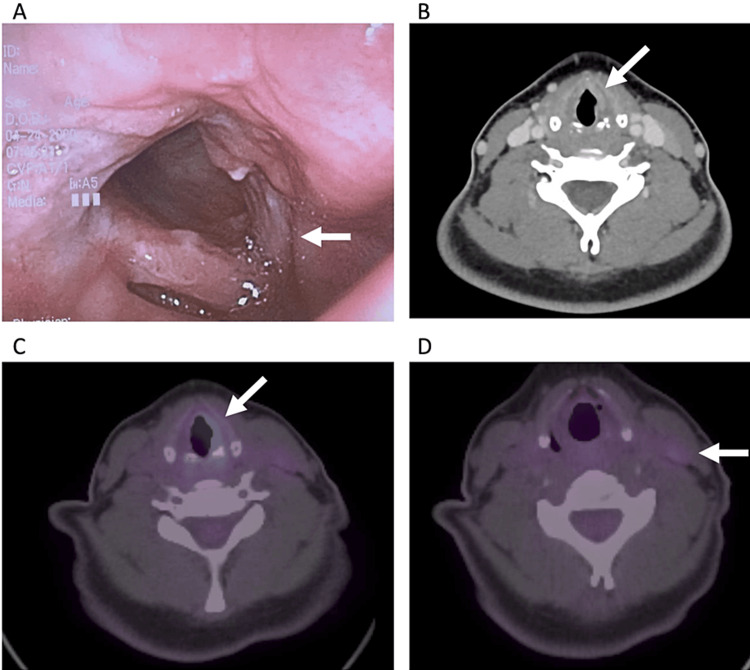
Tumor images obtained for case 2. (A) Naopharyngoscopy demonstrated the left vocal cord lesion (blocked arrow) extending to the anterior commissure. (B) CT imaging of the primary tumor (blocked arrow) with anterior commissure involvement (blocked arrow). PET CT images demonstrating the mildly PET avid primary tumor (blocked arrow) (C) and left neck node (blocked arrow) (D).
